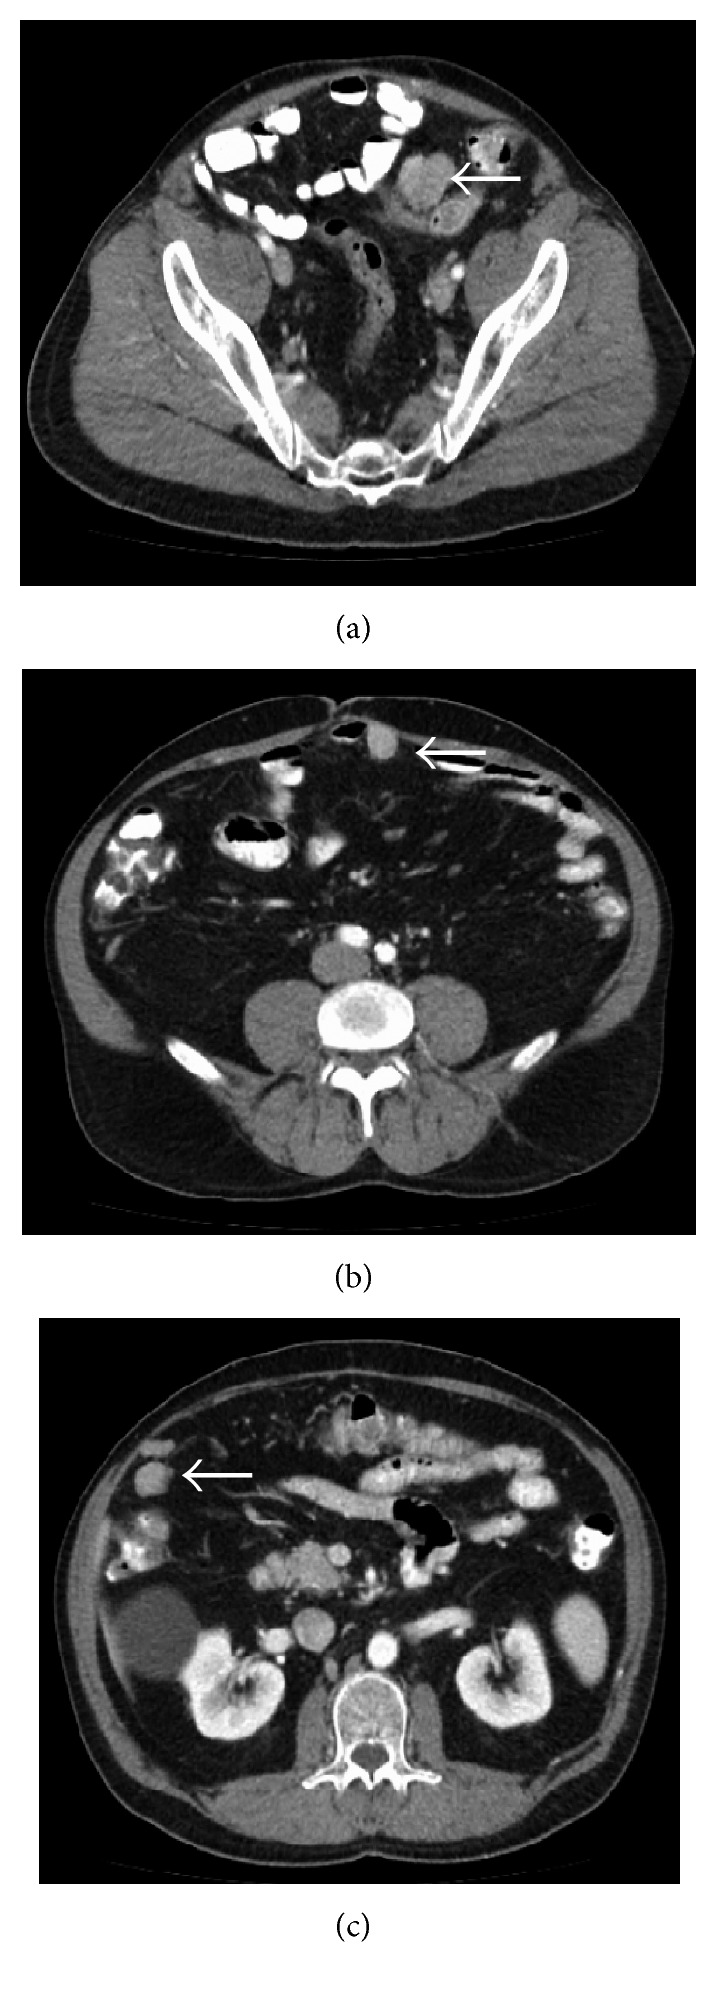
Axial CT images demonstrating recurrent lesions (white arrows) in the left lower quadrant (a), adherent to the anterior abdominal wall (b), and in the right upper quadrant (c).
